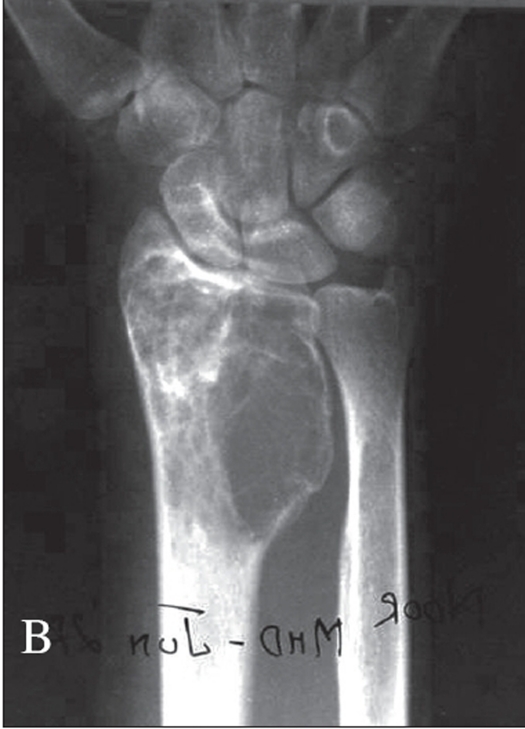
X-ray (antero-posterior) of wrist showing Campanacci grade 2 lesion: Cortical expansion with thin bony rim; but no breach
